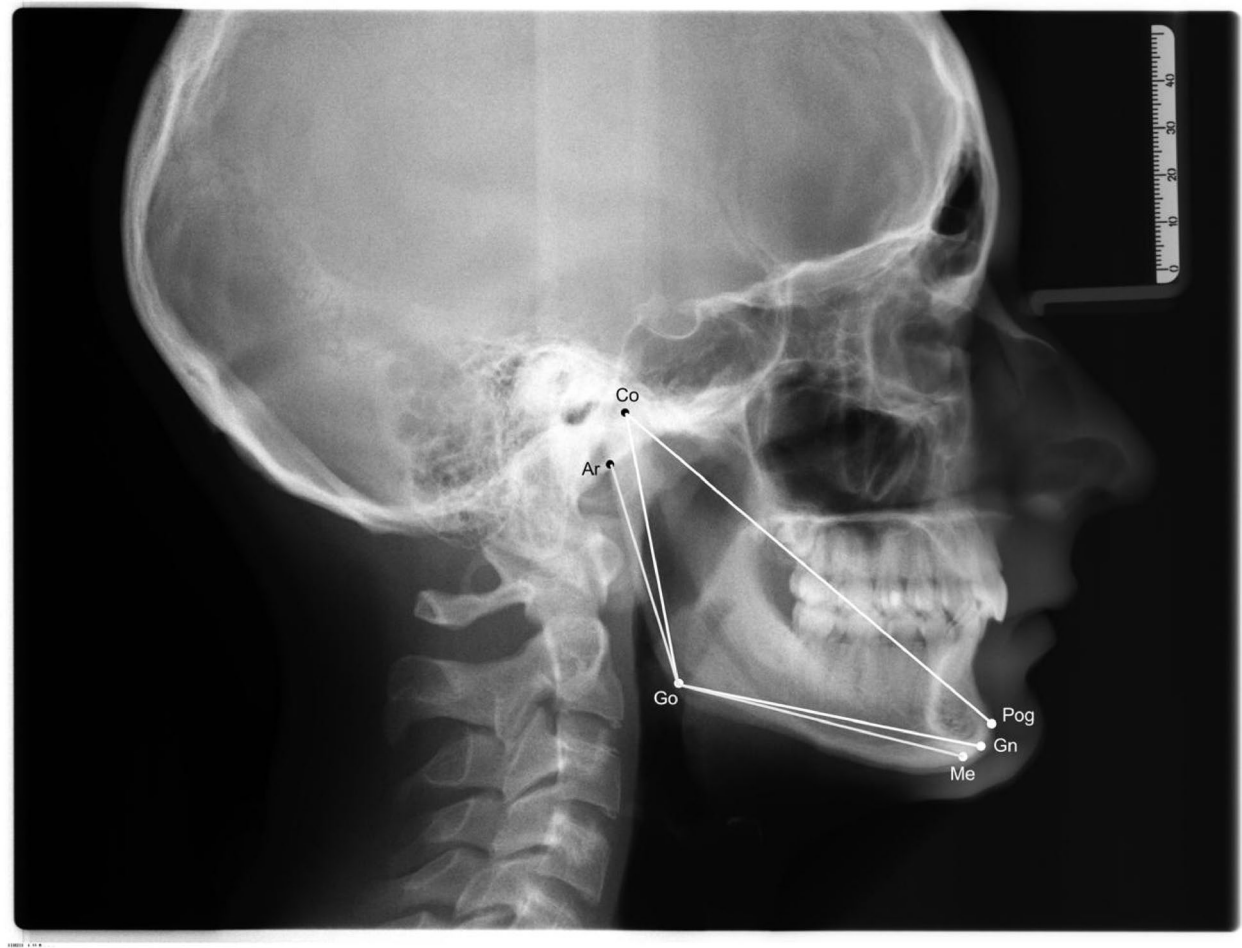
Lateral cephalometric radiograph showing anatomical landmarks. Linear measurements: Co-Pog, Co-Go, Go-Gn. Angular measurement: Ar-Go-Me. GN = Gnathion, ME = Menton (Me), Pog = Pogonion, Go = Gonion; Co = Condylion Ar = Articulare.
